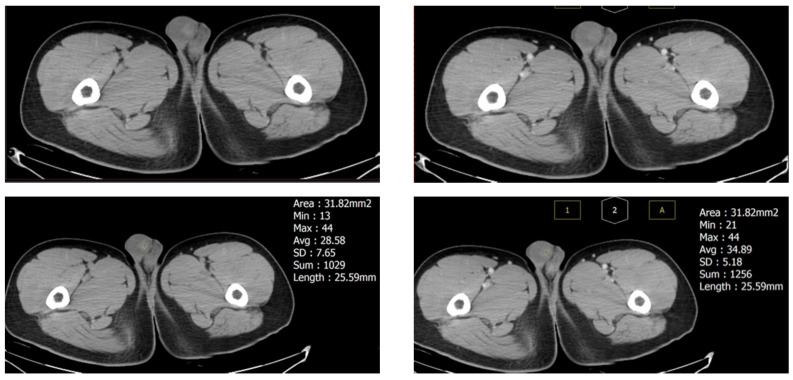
Computed tomography of the testis nine days after the first attack revealed a 13 mm hyperdense mass (32% of testicular volume) without enhancement, which suggests a hematoma. The size of the testis had decreased compared to the first attack.
